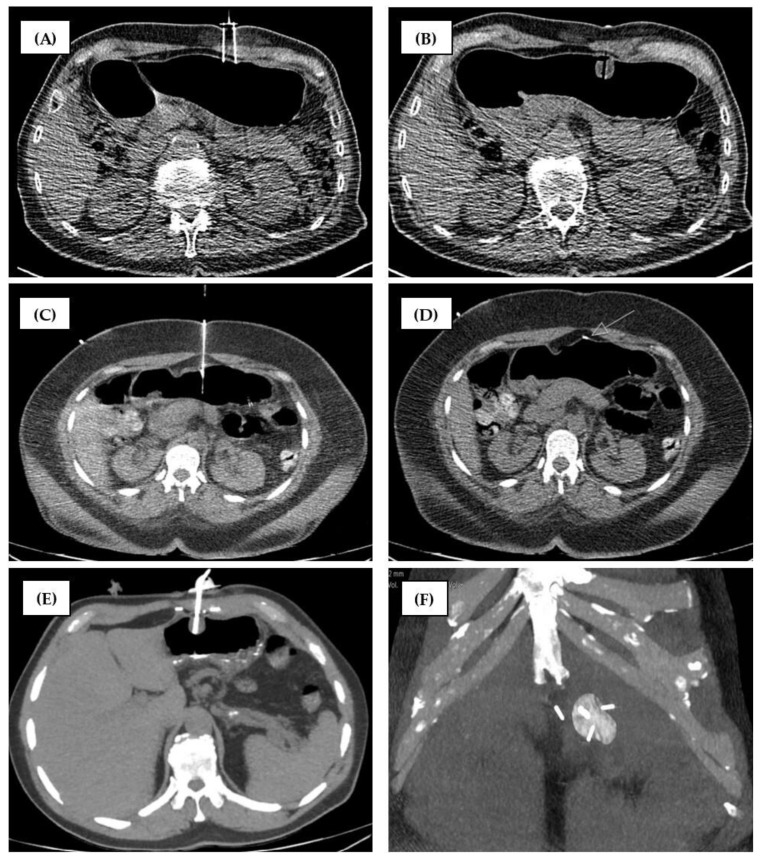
Comparison of fixation techniques. (A,B) technique of center 2. (C–F) technique of center 1. (A) Gastropexy Device II (Fresenius Kabi, Bad Homburg vor der Höhe, Germany) with two parallel hollow needles and a thread inside. (B) After gastropexy, the suture is not visible on CT. (C) A first SAF-T-PEXY T-Fastener anchor (Avanos Medical, GA, USA) was placed in the body of the stomach. (D) The anchor is visible in the CT. The arrow shows the correct end position on the inner stomach wall. (E) Gastric tube placed between the anchors. (F) The distances between the anchors are different. As usual, the tube was placed in the center of the triangle.
